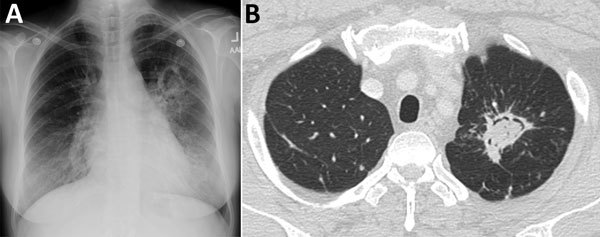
A) Chest radiograph showing aspergilloma (fungal ball) in the left upper lung lobe. B) Axial computed tomography image shows increased density in an irregular left apical cavity, a sequela of pulmonary tuberculosis, consistent with an aspergilloma.
